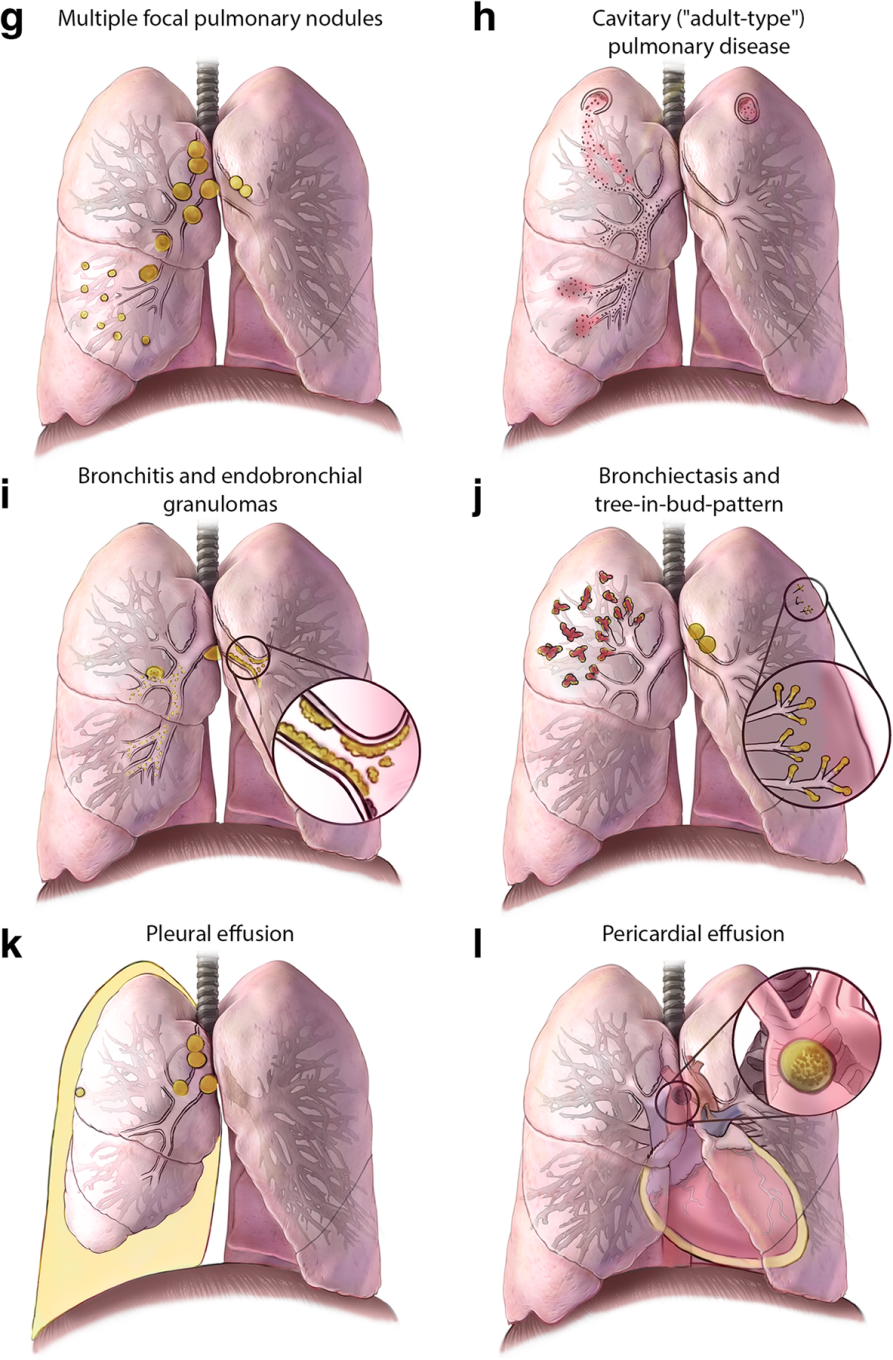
Panel g. Multiple focal pulmonary nodules. Multiple focal pulmonary nodules involving the right middle lobe with enlargement of regional lymph nodes (*right lung*). Panel h. Cavitary (“adult-type”) pulmonary disease. Cavity formation in both upper lobes, with endobronchial spread to the right middle lobe. Nodules or cavities in apical lung segments are typical of adult-type disease and are pathologically distinct from the other cavities shown. Panel i. Bronchitis and endobronchial granulomas. Inflammation of the mucosa of main stem bronchus with purulent secretions (*left lung*), and a necrotic lymph node that has eroded into the right middle lobe bronchus leading to endobronchial spread and subsequent development of endobronchial granulomas extending proximally to the bronchus intermedius and main stem bronchus, and distally to the lower lobe bronchus (*right lung*). These findings are best visualized by bronchoscopy. Panel j. Bronchiectasis and tree-in-bud-pattern. Bronchiectasis that extensively involves the upper lobe (*right lung*), and shows tree-in-bud pattern observable on CT scans -- reflecting dilated centrilobular bronchioles with mucoid impaction -- involving the upper lobe (*left lung*). Panel k. Pleural effusion. A pleural effusion that is usually indicative of recent primary infection, with a hypersensitivity response to tuberculoprotein leaking from a subpleural Ghon focus (often not visible) into the pleural cavity; in rare cases this effusion may also result from a chylothorax. Panel l. Pericardial effusion. A pericardial effusion that occurs when tuberculoprotein leaks from a necrotic subcarinal lymph node (shown in “close-up” window) into the pericardial space; it may also occur after hematogenous spread. Conceptualization and original sketches by C.L. Roya-Pabon, MD; finished artwork by Mesa Schumacher, MA (used with permission). Adapted from C.M. Perez-Velez. Diagnosis of Intrathoracic Tuberculosis in Children. In: Handbook of Child and Adolescent Tuberculosis (p. 154–155), J.R. Starke and P.R. Donald (Eds.), 2016, New York, NY: Oxford University Press. Copyright by Oxford University Press [15]. Adapted with permission
